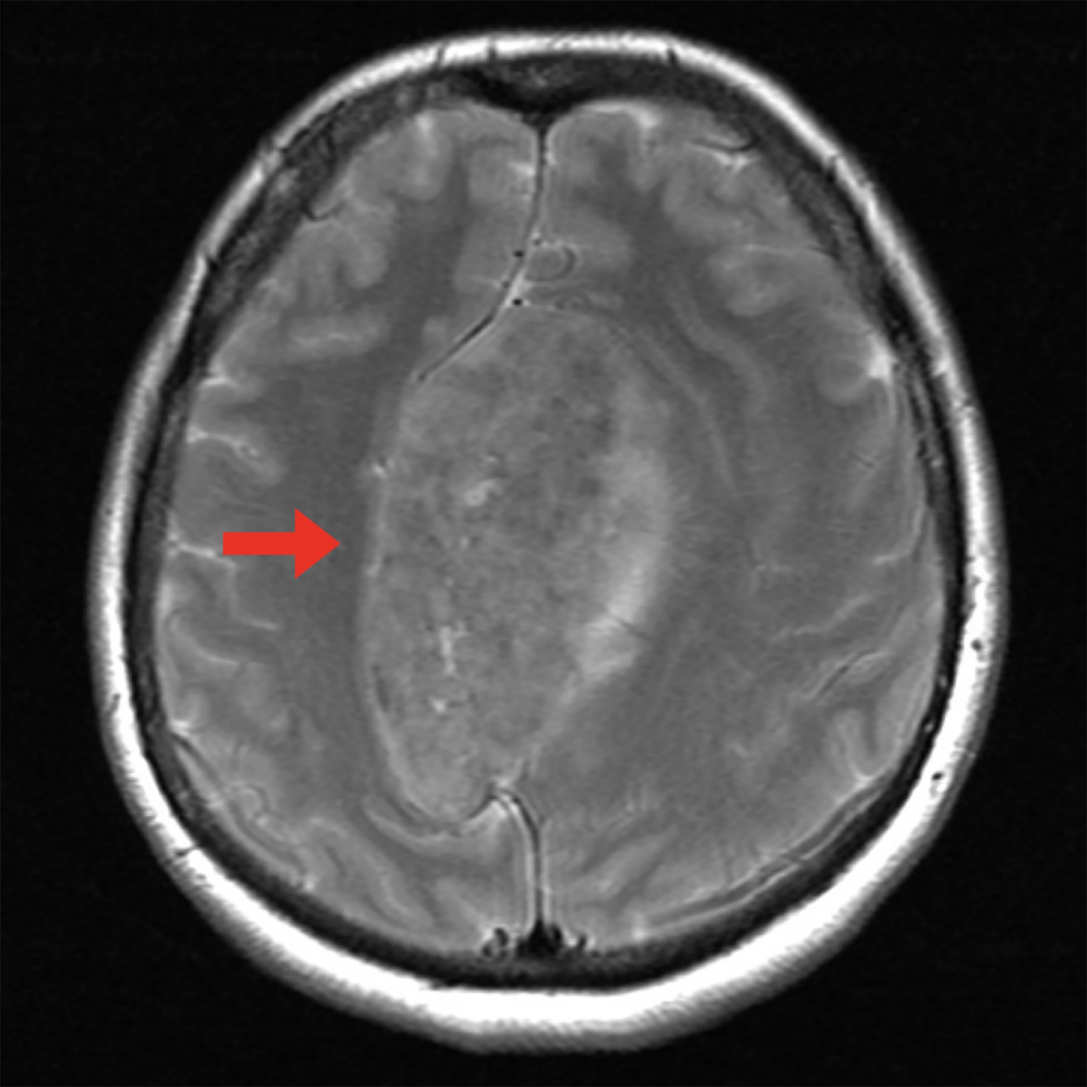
Preoperative T2-weighted magnetic resonance imaging (MRI) Axial T2-weighted MRI demonstrating lack of edema in peritumoral brain (red arrow).
